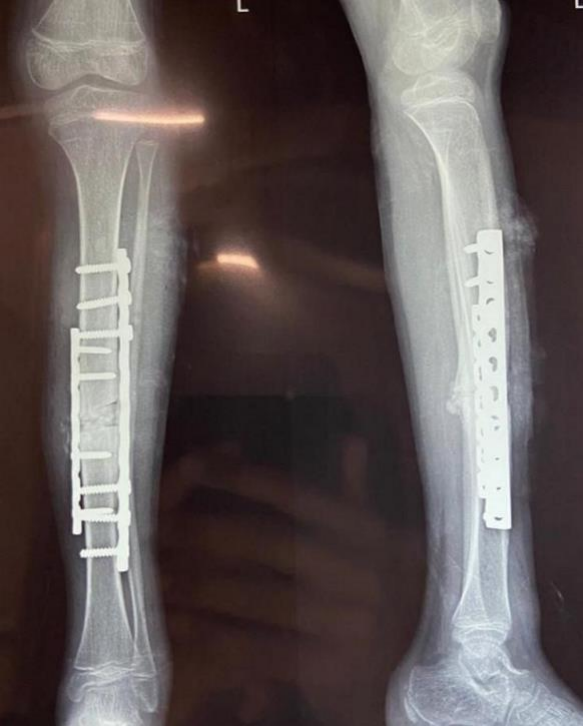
postoperative X-ray showing open reduction and internal fixation with plate osteosynthesis and bone grafting for midshaft tibia fracture
